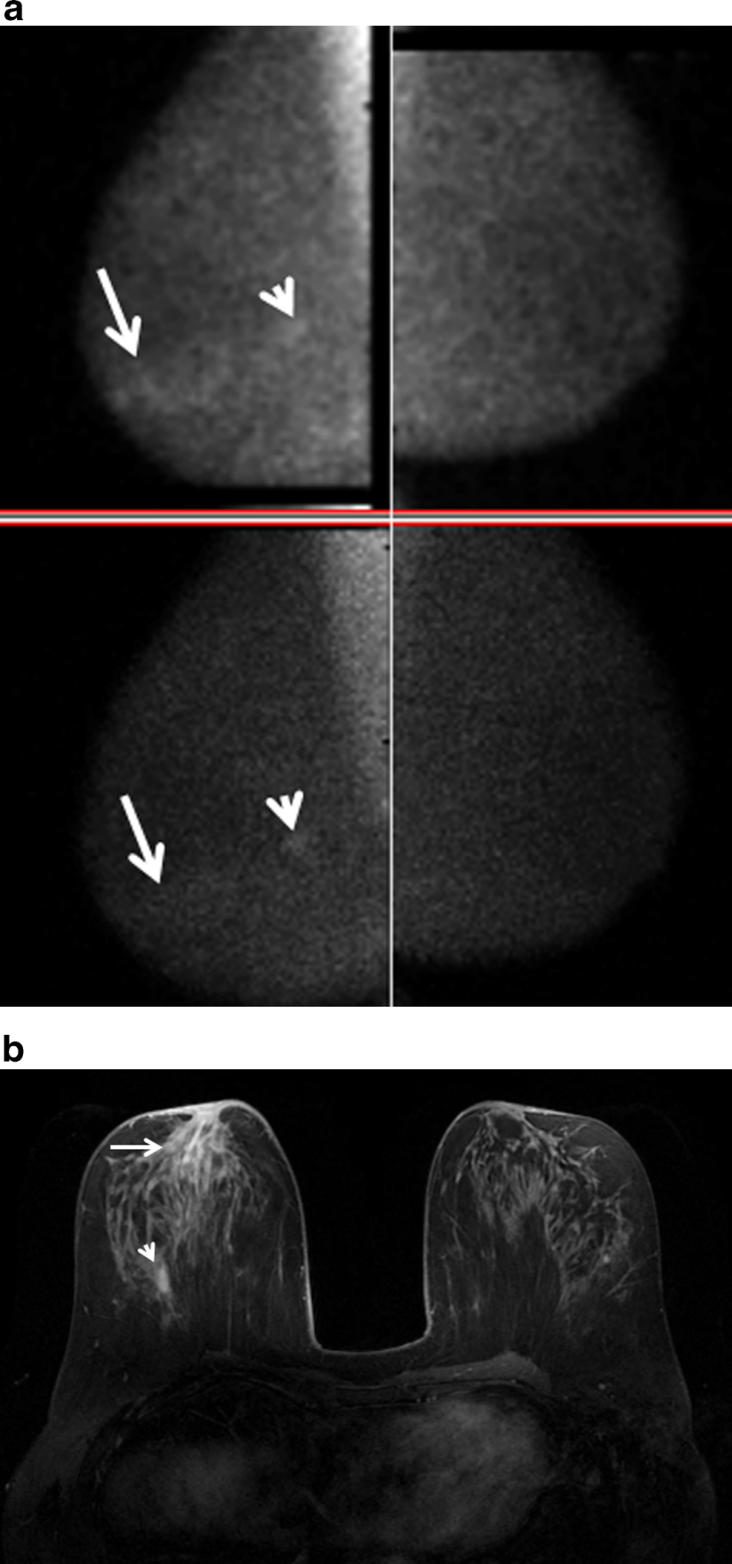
A 50-year-old woman with right breast pain and redness not resolving with antibiotics. Biopsy showed IGM. a Dual head prototype MLO bilateral molecular breast imaging (MBI) scanned prior to commercially available MBI demonstrates mild unilateral right non-mass subareolar uptake (*arrows*) best seen on the superior detector (*upper left image*). The posterior area of mass enhancement (*arrowhead*) was a biopsy proven fibroadenoma. Left breast is negative. b Axial T1 weighted post gadolinium fat saturated MRI image shows non-mass enhancement in the right subareolar region corresponding to the focal uptake on MBI (*arrows*); MR-guided biopsy revealed IGM. The enhancing fibroadenoma is noted posterior depth right breast (*arrowhead*)
